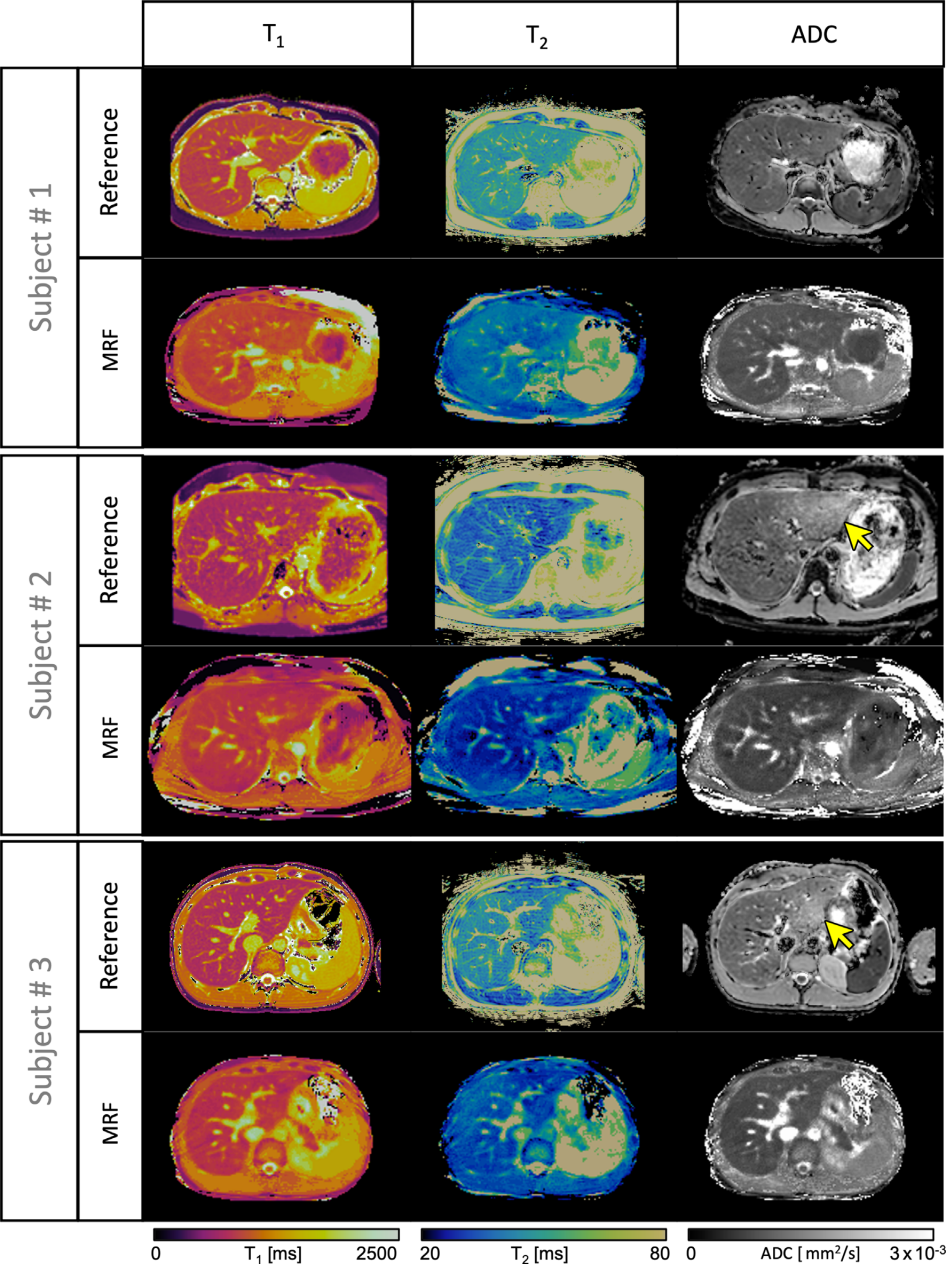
Abdominal axial views of T_1_, T_2_, and ADC quantitative maps in three representative healthy subjects. Reference maps (top row for each subject) of T_1_‐MOLLI, T_2_‐GRaSE, and SE‐DWI are compared against the proposed simultaneous T_1_, T_2_, and ADC MRF maps (bottom row for each subject). Yellow arrows point to abnormally elevated ADC values on reference maps (known artifacts due to cardiac motion), that are not observed in MRF
